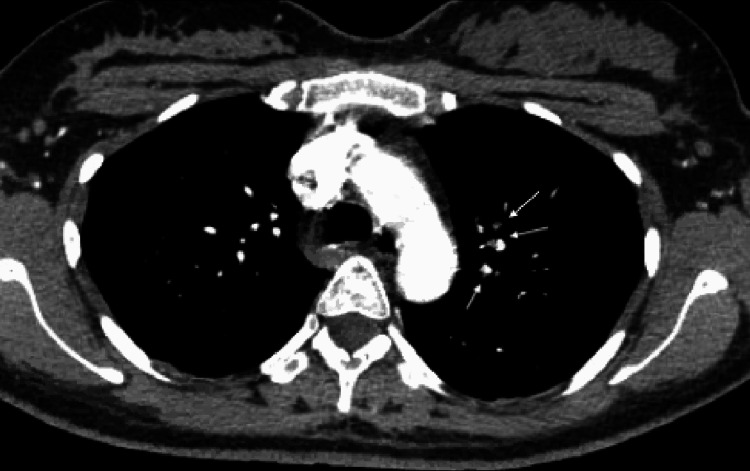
Axial view of CTPA revealing filling defects in the left upper lobe. CTPA: computed tomography pulmonary angiogram
